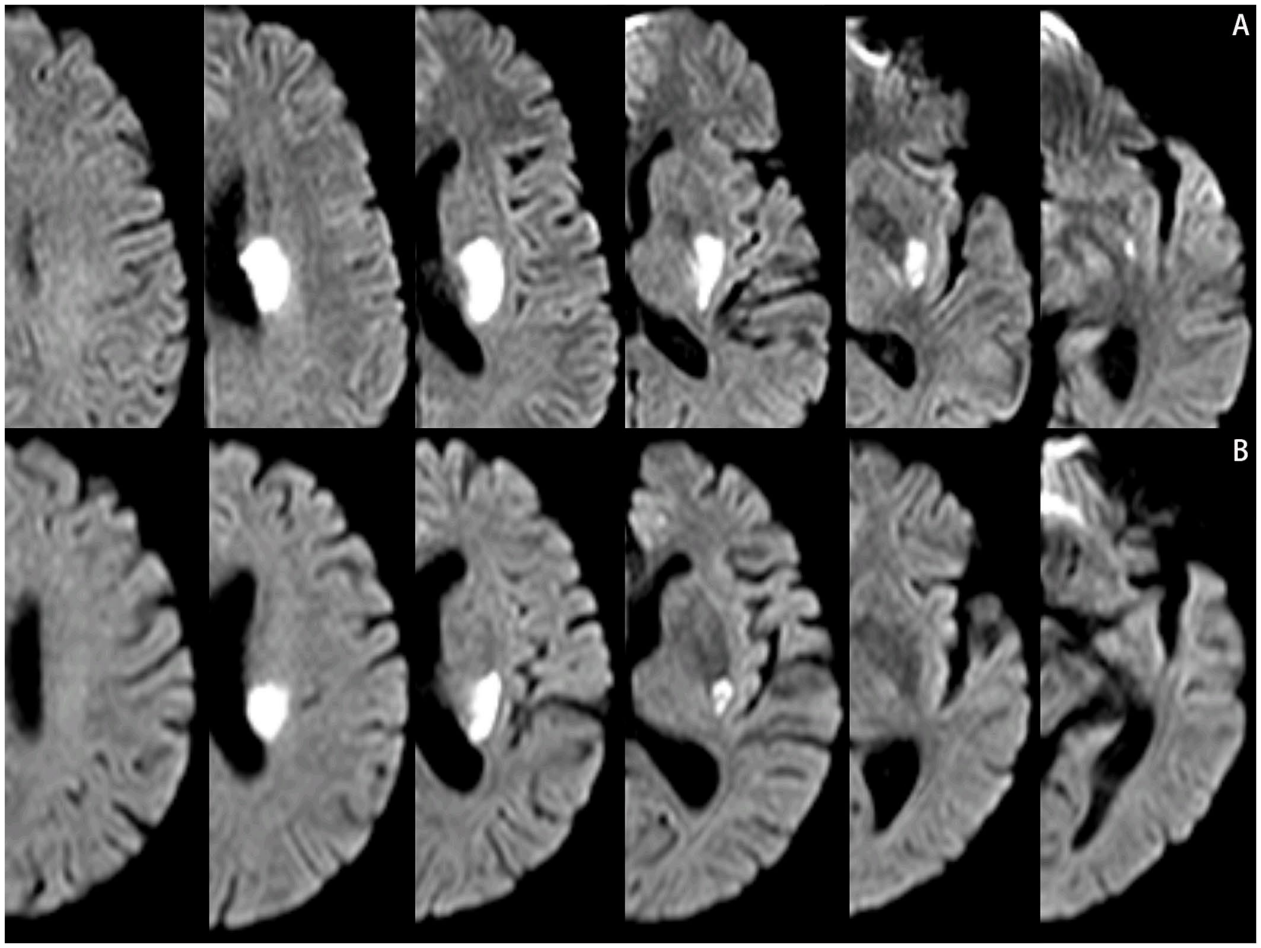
Lesions location on the diffusion-weighted MRI. (A) Proximal single subcortical infarction. (B) Distal single subcortical infarction.
